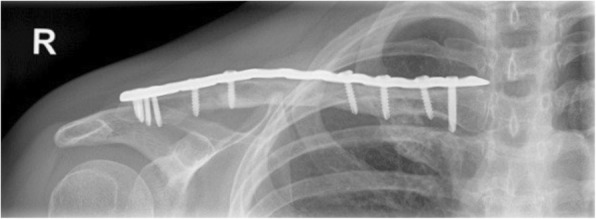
Postoperative AP X-ray of the clavicle in case 1
